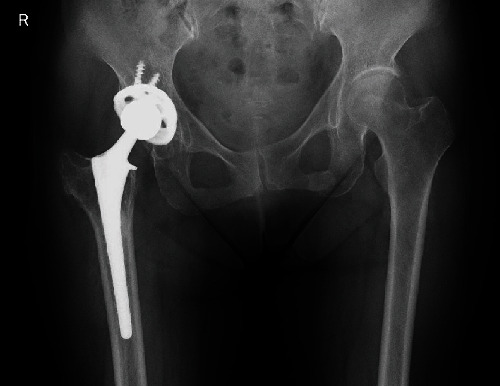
Radiographs of Case 1 last follow-up (7 years) after total hip arthroplasty.
